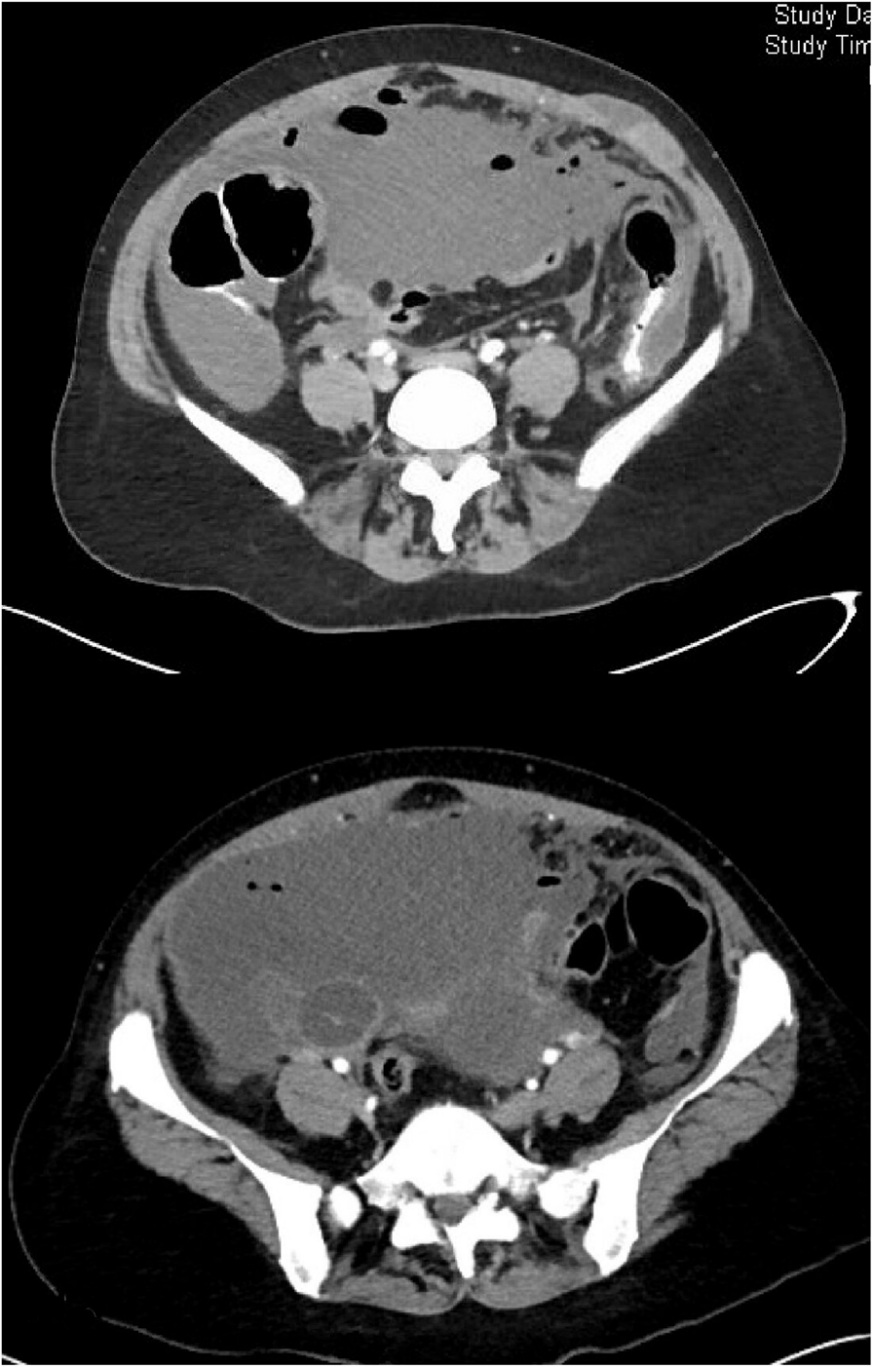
Representative images of CT of the abdomen/pelvis upon presentation with moderate-volume ascites, complex fluid within the abdomen and pelvis with extensive gas
